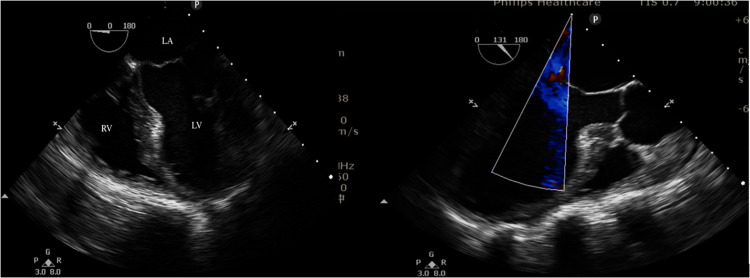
Intraoperative transesophageal echocardiograms.
